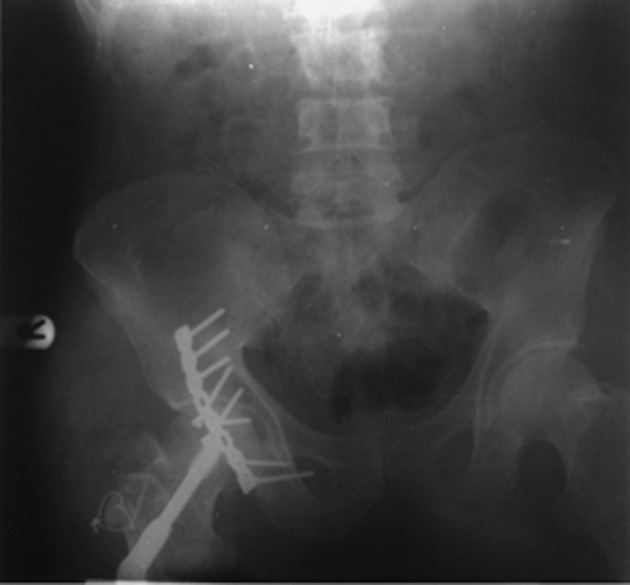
Radiograph After Eight Months
